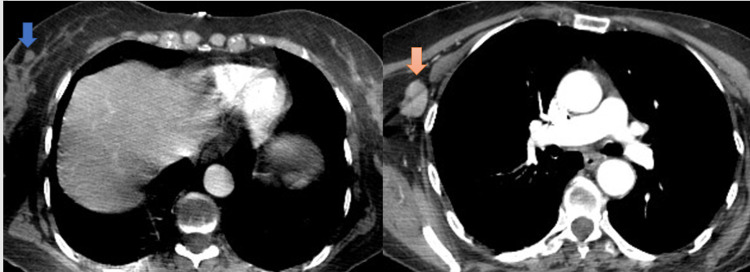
Chest CT scan with IV contrast shows right axillary lymphadenopathy IV: Intravenous; CT: Computer tomography Left-hand side figure panel: the blue arrow shows a small right breast nodule measuring 7.4 mm on the short axis Right-hand side figure panel: the peach-colored arrow shows right axillary lymphadenopathy measuring 25.7 mm on the long axis and 16.3 mm on the short axis
